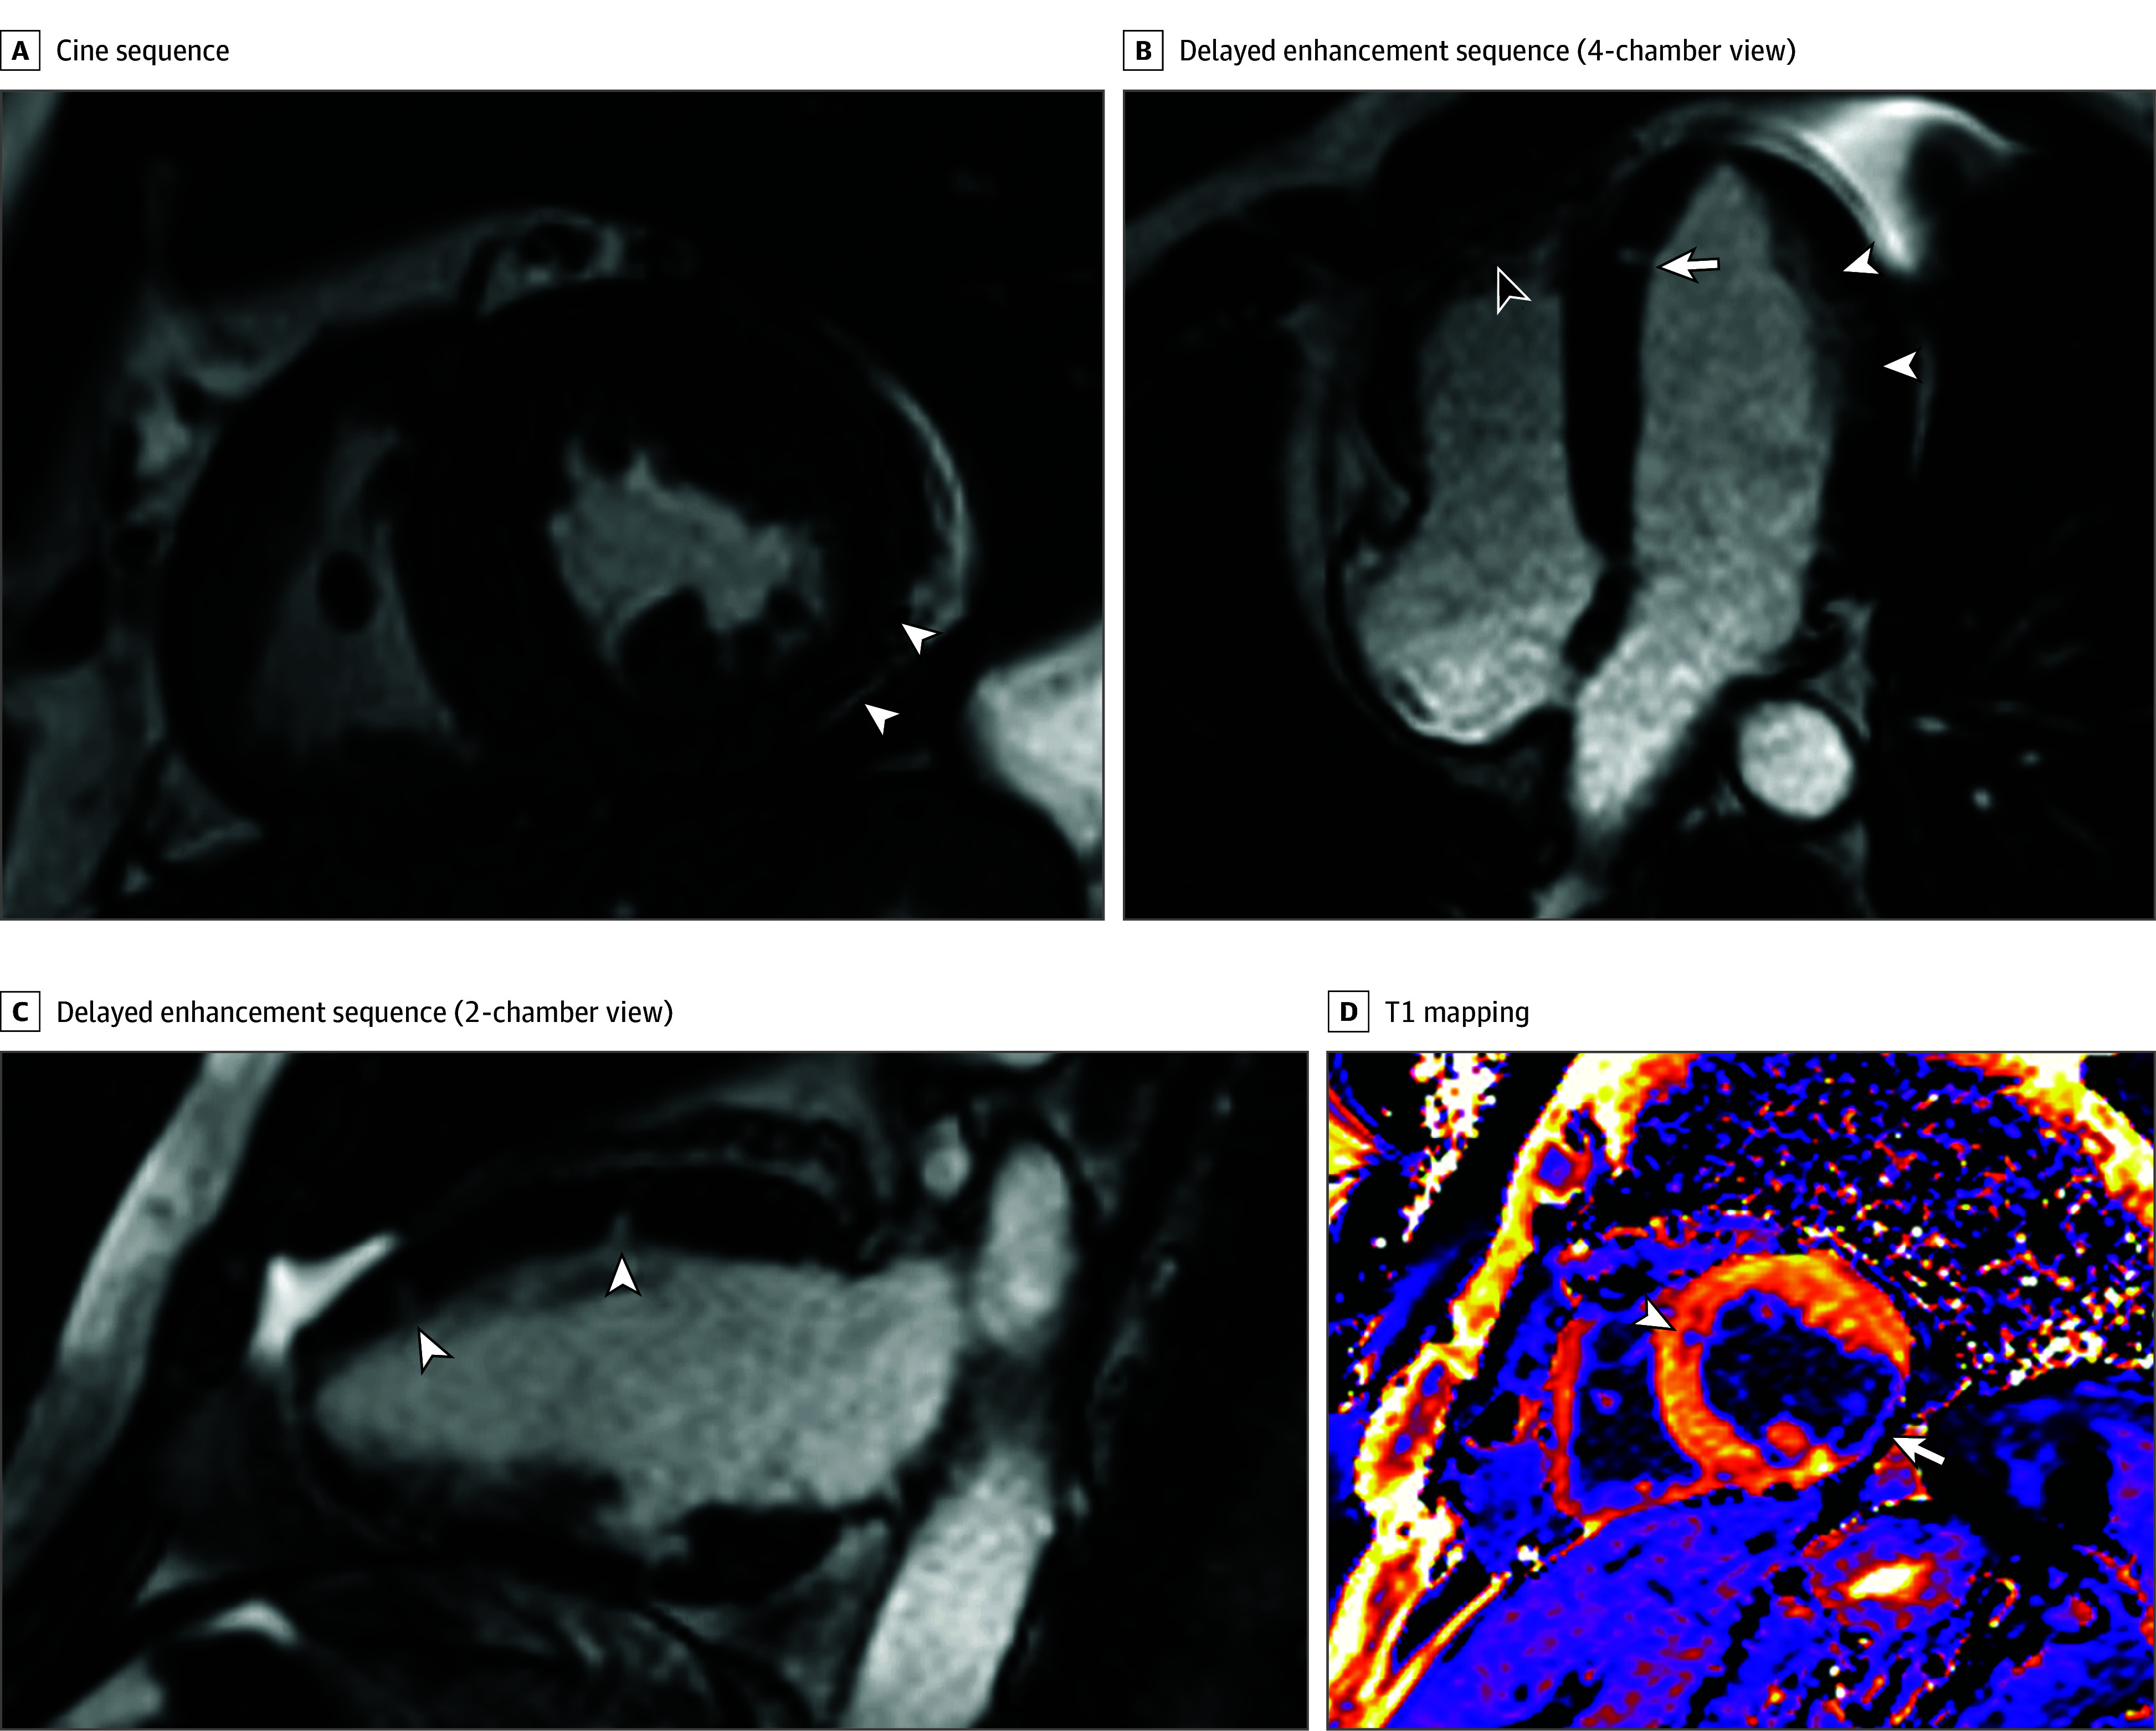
Cardiac Magnetic Resonance Imaging of Patient 3 Showing Myocardial Infarctions and Necrosis Sequalae A, Cine sequence, with midventricular short-axis view at end systole showing severe thinning of the inferolateral wall of the left ventricle (white arrows). B, Delayed enhancement sequence performed 10 minutes after gadolinium injection (4-chamber view), with subendocardial enhancement of the left ventricular wall (white arrowheads), nodular enhancement of the apical septum (white arrow), and enhancement of the right ventricular apex (black arrowhead). C, Delayed enhancement sequence performed 10 minutes after gadolinium injection (2-chamber view), with nodular foci of enhancement of the anterior wall of the left ventricle (arrowheads). D, T1 mapping performed 12 minutes after gadolinium injection, with midventricular short-axis view showing decreased signal of the inferolateral wall of the left ventricle (arrow) due to gadolinium retention and nodular focus of decreased signal in the anterior septum (arrowhead).
